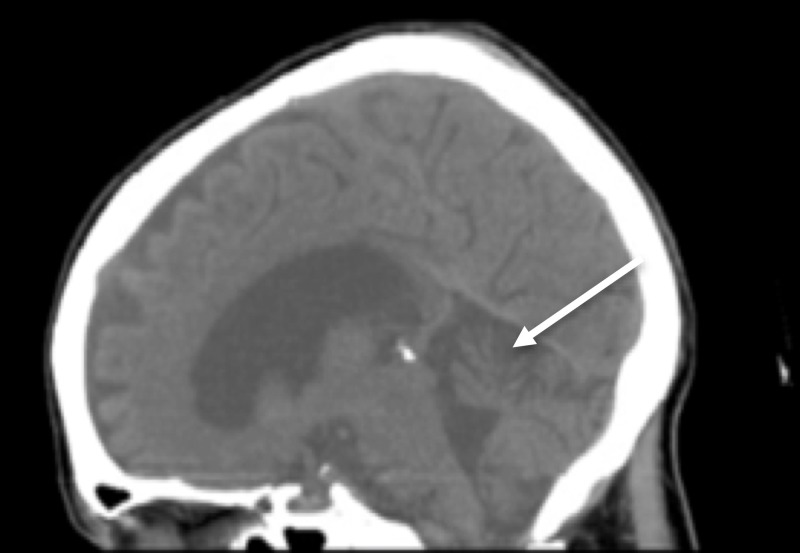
Midsagital CT of the head Midsagital CT of the head shows cerebellar atrophy, most pronounced in the vermis (arrow)
